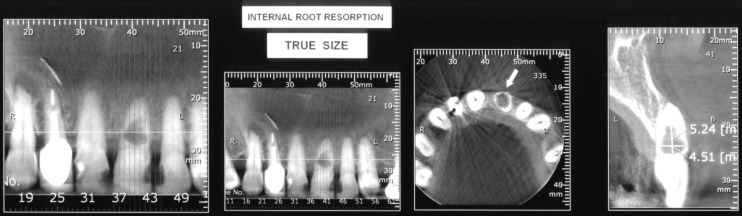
CBCT image showing the extent of internal inflammatory root resorption in the maxillary left central incisor
